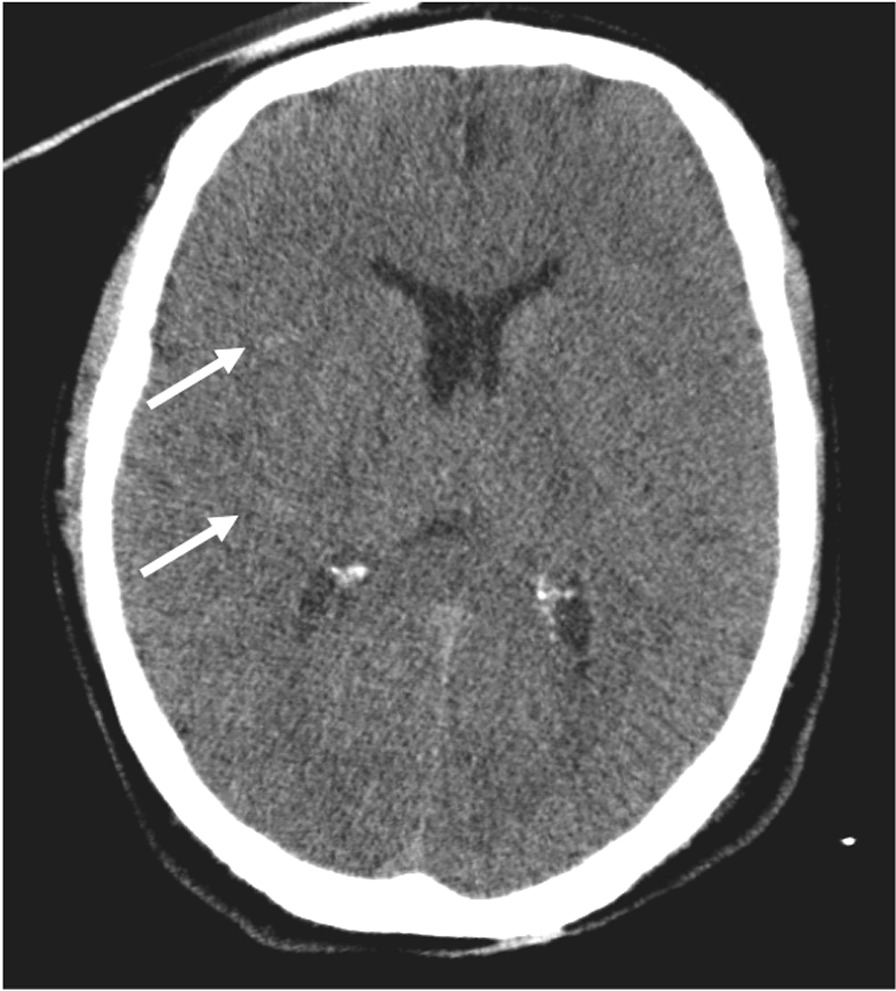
Axial non-enhanced brain computed tomography scan demonstrates a loss of grey-white matter differentiation at the fronto-temporal lobes bilaterally with gyral effacement. Subtle subarachnoid hemorrhage (white arrows) is also noted
